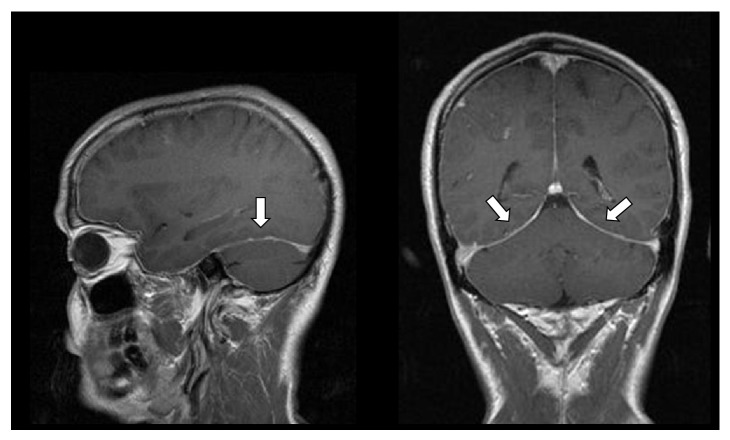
Brain MRI. Diffuse pachymeningeal enhancement after gadolinium administration (arrows), suggestive of liquoral hypotension.
